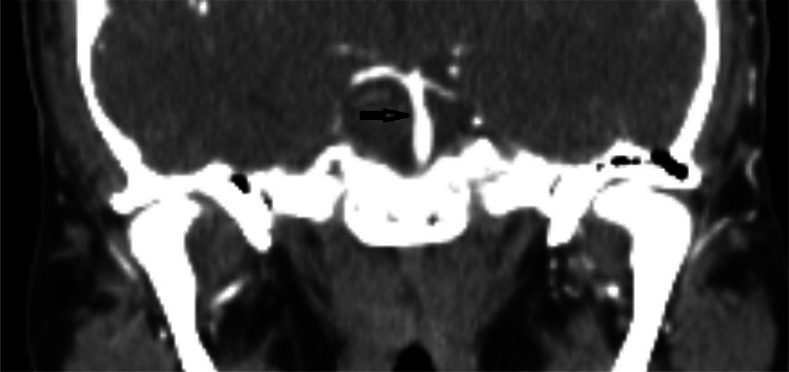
CTA coronal section 24 days post-diagnosis.
